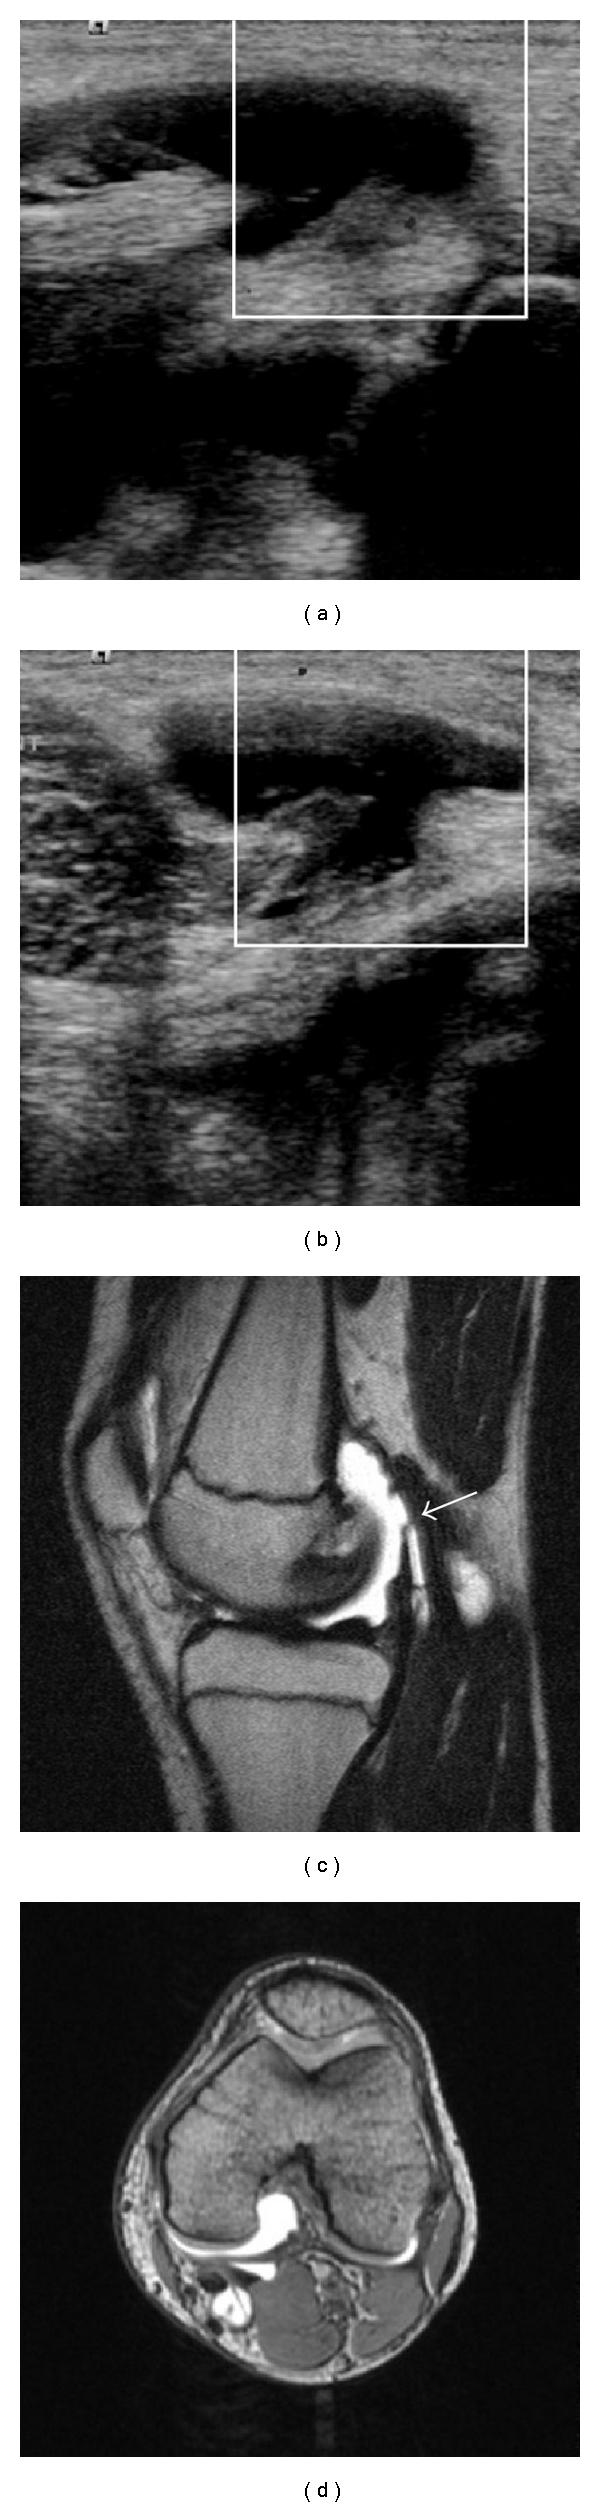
Baker cyst of the left knee in a 14-year-old girl with juvenile rheumatoid arthritis. Ultrasonography demonstrated the cystic lesion with 2.8 mL volume and echogenic sedimentation (a,b). Magnetic resonance imaging (1.5 Tesla MAGNETOM Symphony, Siemens, sagittal proton-density and T2-weigthed imaging, transversal DESS sequence) depicts the cyst with homogenous liquid signal and suggests continuity between cyst and joint space in the presence of joint effusion.
